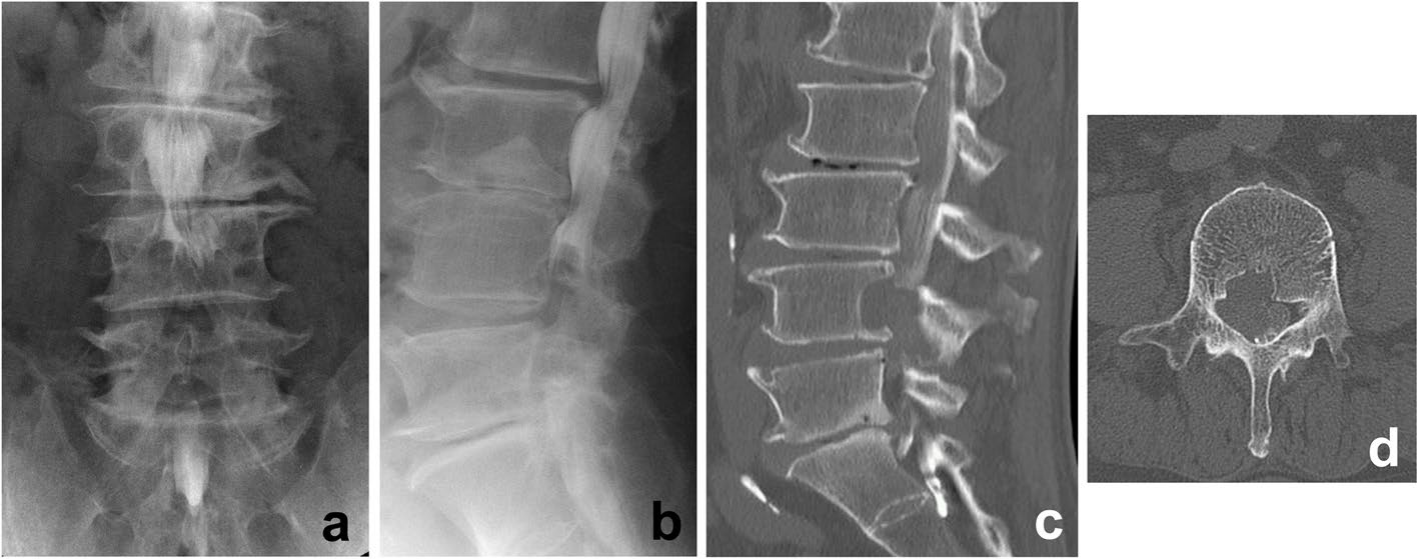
X-ray and computed tomography (CT) findings. a Anteroposterior and (b) lateral preoperative X-ray images (obtained following myelography) of the lumber spine, revealing the right-side dominant contrast defect of the spinal subarachnoid space at the L3–4 level. c CT images of the right parasagittal and (d) axial aspects at the level of the L4 vertebra (following myelography), revealing severe scalloping at the right side of the L4 vertebral posterior wall
